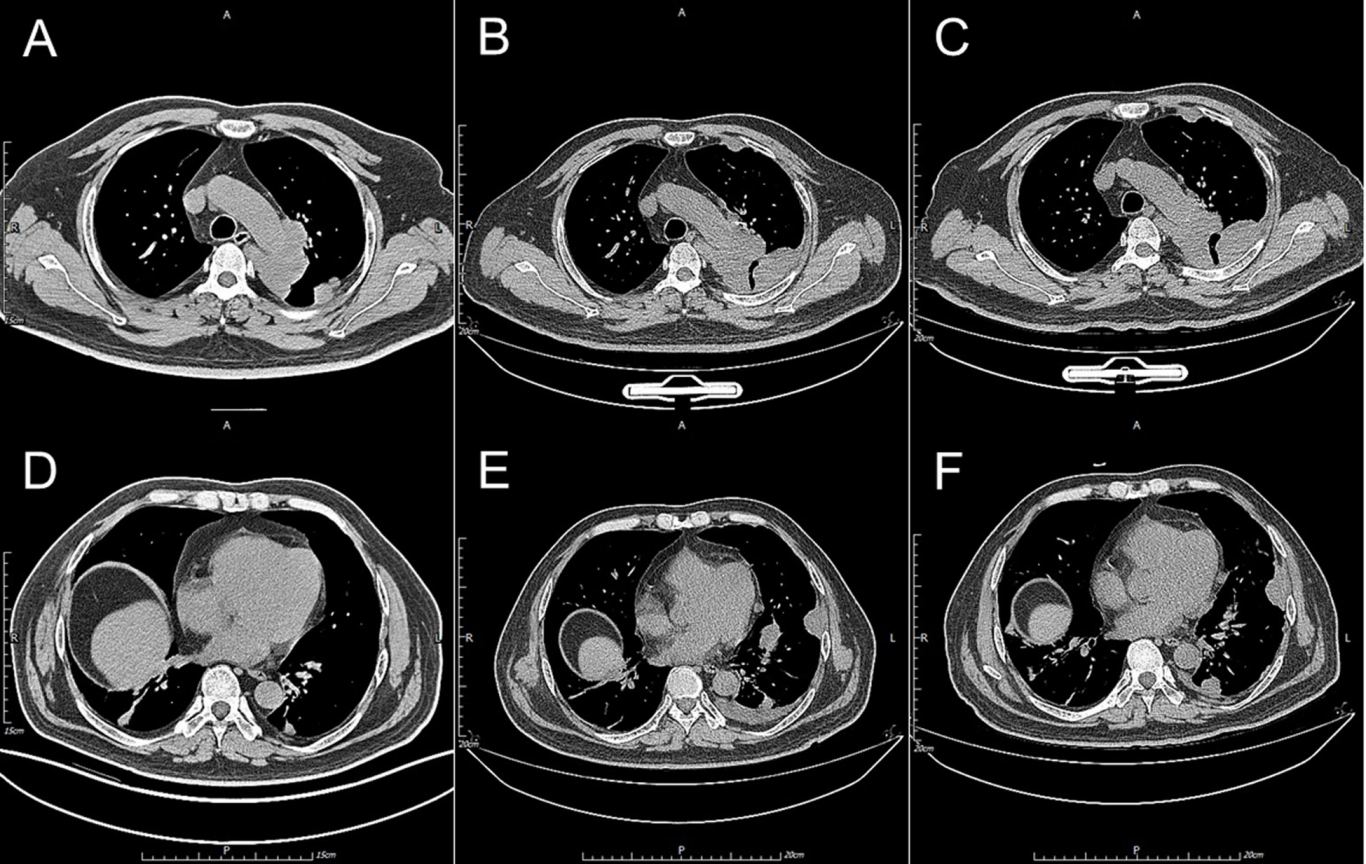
(A) CT scan of the patient with PEA throughout his treatment is presented. Images (A, D) acquired on June 22nd, 2024, demonstrate a mass adjacent to the right pulmonary hilum with evidence of pleural invasion. Despite undergoing a series of treatments, the mass exhibited rapid growth over a two-month period [images (B, E)]. Notably, following the initiation of ALK-targeted therapy for 2 months, the tumor demonstrated disease stabilization and partial regression [images (C, F)].
